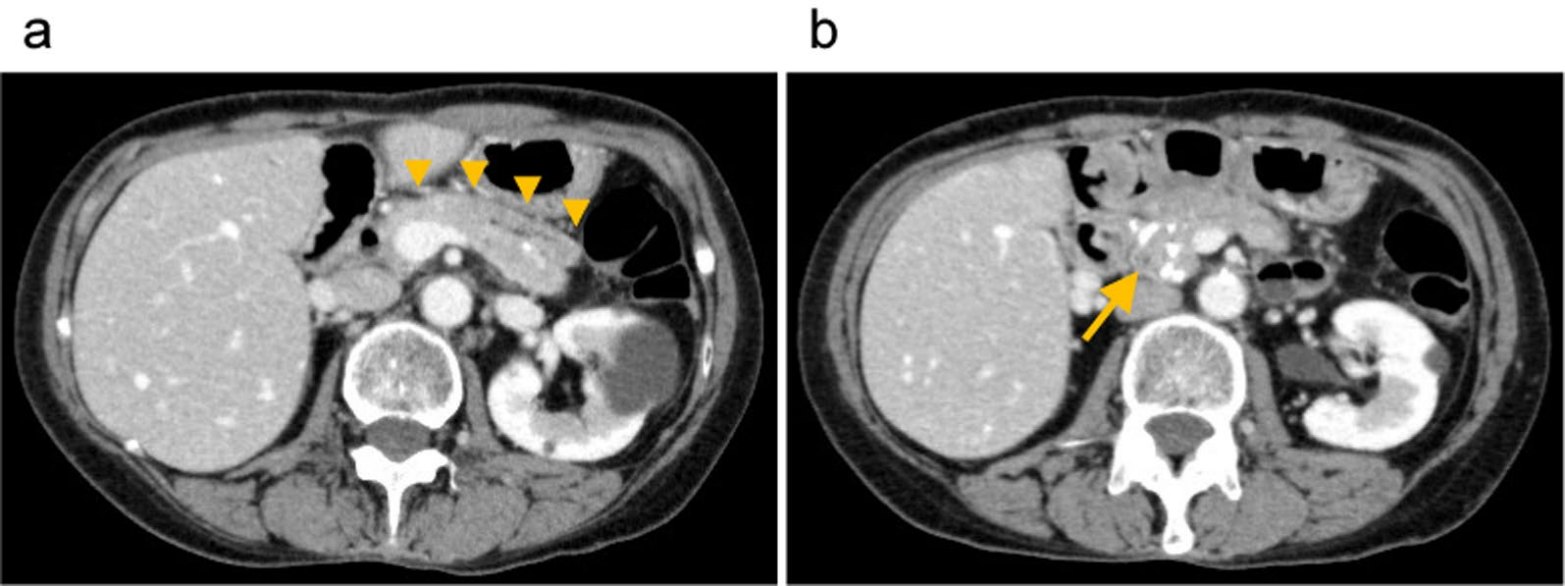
Preoperative abdominal computed tomography imagings. a Preoperative abdominal computed tomography imaging showing longitudinal pancreaticojejunostomy performed in the previous Frey’s procedure (arrowheads). b Preoperative abdominal computed tomography imaging showing multiple pancreatic calculus in the pancreatic head (arrow)
